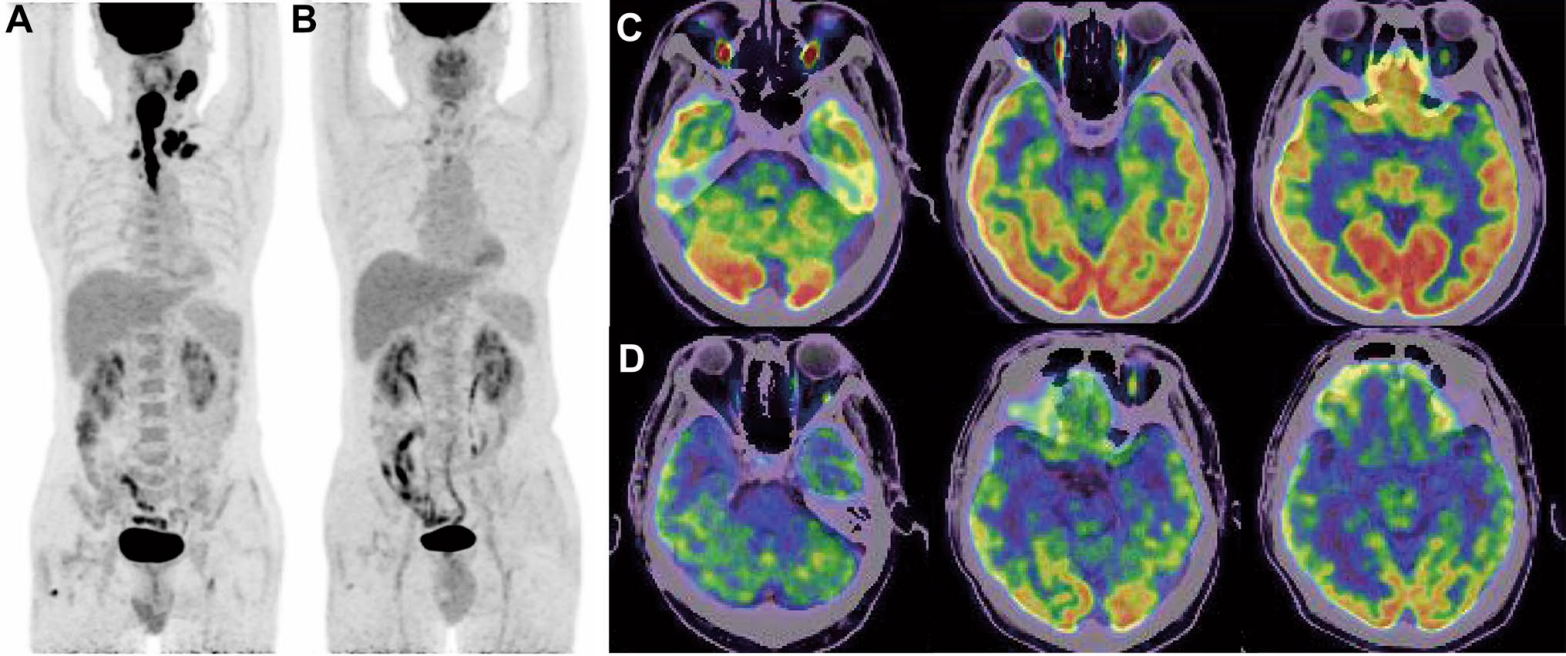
The imaging changes of PET/CT before and after four cycles of Camrelizumab plus albumin paclitaxel combined with cisplatin. A Whole-body PET/CT showed that the patient’s primary lesion and metastasis in lymph nodes. B Whole-body PET/CT showed the patient achieved PR after four cycles of immunotherapy with chemotherapy. C and D PET/CT showed that corresponding areas were normal before and after four cycles of immunotherapy with chemotherapy
